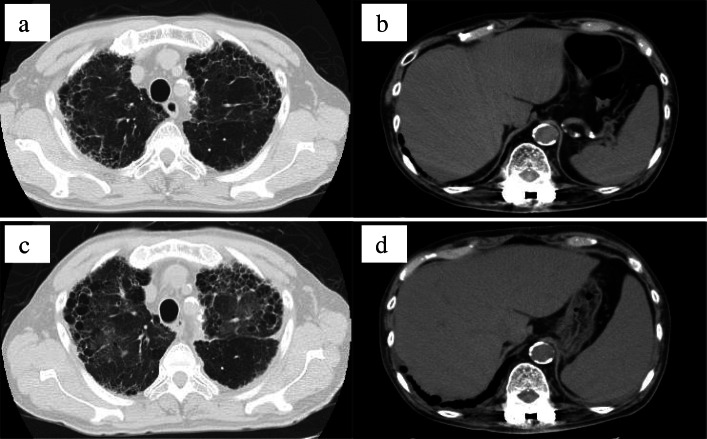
Computed tomography (CT) scan of interstitial pneumonia (IP) and hepatosplenomegaly. IP with reduced activity (a), and normal-size liver and spleen on day 1 (b). Acute exacerbation of IP with expanded ground-glass shadow in both lung areas on day 20 (c). Aggressive hepatosplenomegaly on day 20 (d)
